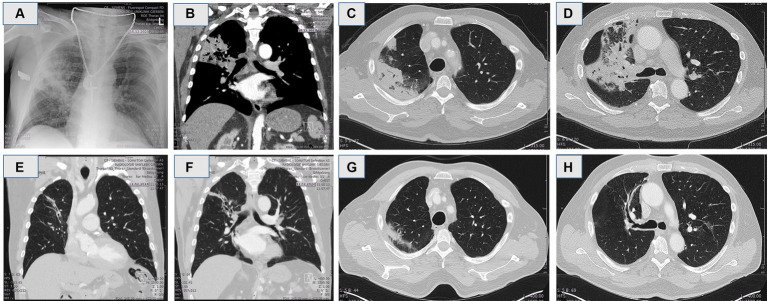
(A) Chest X-ray showing a consolidation in the right upper lobe indicating of pneumonia. (B–D) Computerized tomography (CT) in coronal and axial reconstruction demonstrated compression of the upper right bronchus and post-stenotic pneumonia of right upper lobe resembling lung cancer. (E–H) Computerized tomography in coronal and axial reconstruction 8 weeks post extraction of the cherry pit.
